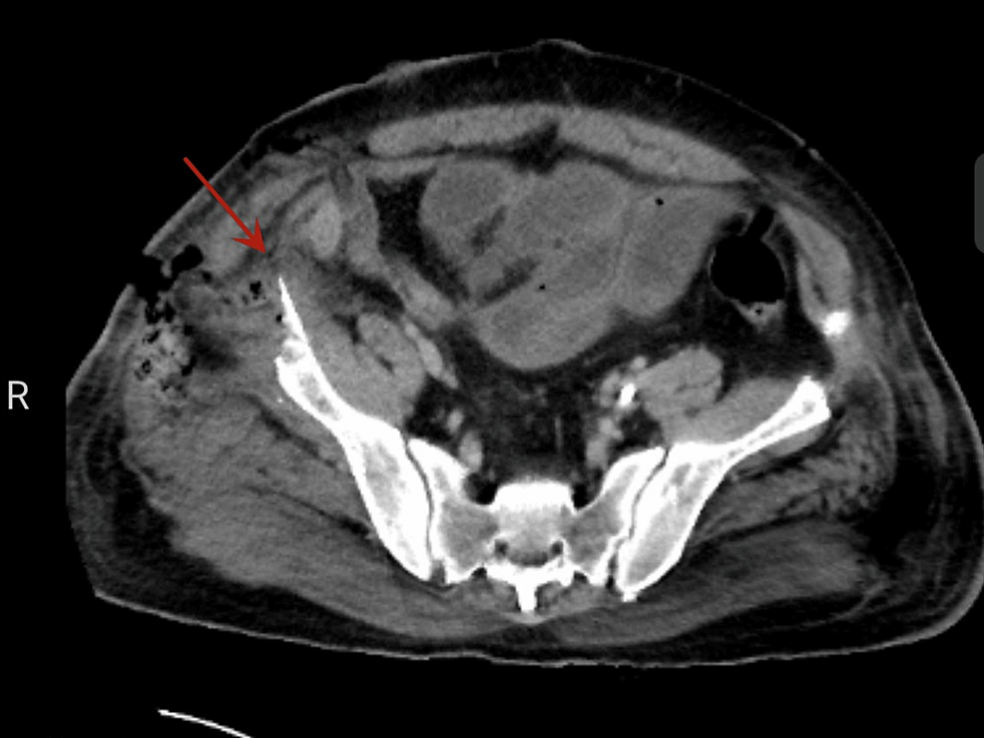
Abdominal CT scan (axial section) showing that there is gas in the right lower abdomen with the possibility of faecal accumulation
